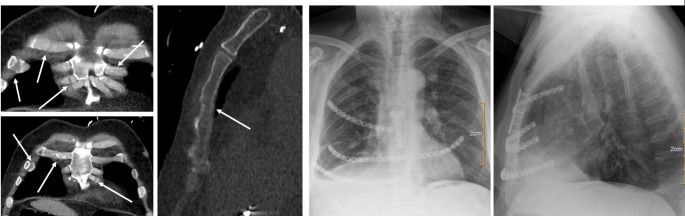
The typical CT-scan findings are usually difficult to find, since the sternal fracture is undisplaced and the osteochondral dissociation needs special attention. The surgical strategy addresses the injury pattern: plating osteosynthesis of the sternum + transsternal fixation of at least the 5th rib on both sides. If additional fractures are displaced in the anterior or antiolateral column, additional plating can be beneficial as seen in the postOP x-ray
